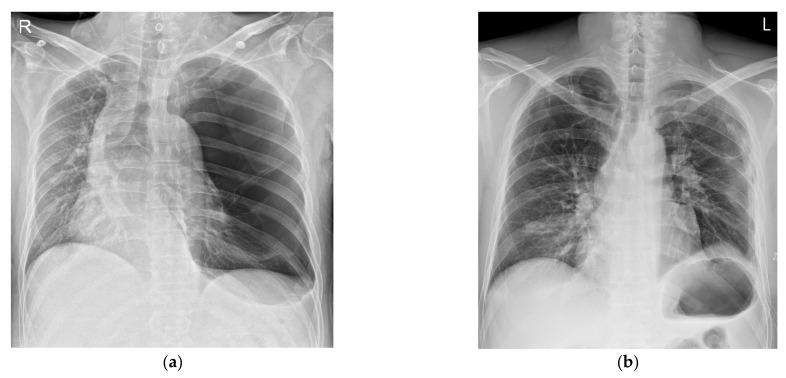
Chest X-ray at the time of admission (a) and on the first day after the operation (b). It can be seen that the left lung collapsed, and the mediastinum shifted to the right (a).
